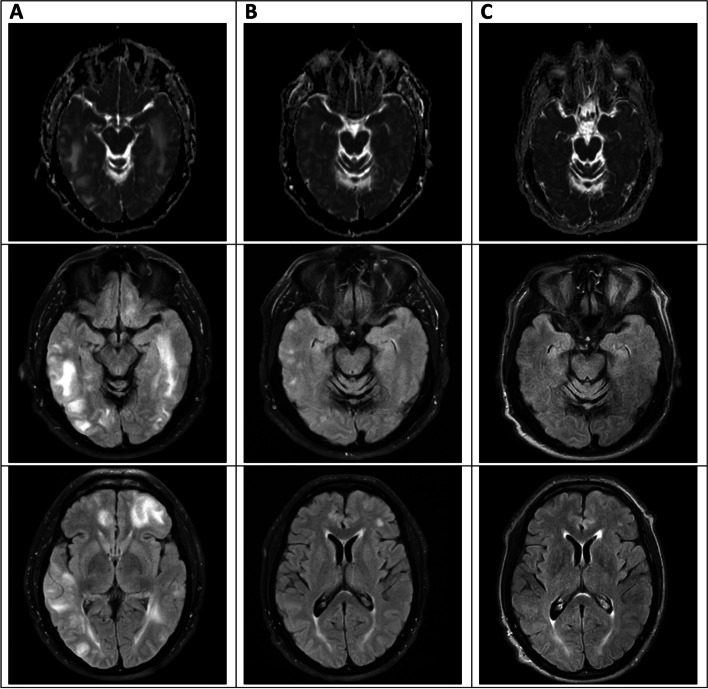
MRI sequences related to patient’s PRES. Top row Diffusion, Middle and Bottom Row FLAIR (A) Initial Presentation (B) 1-month after stopping Mepolizumab (C) 6-months after stopping Mepolizumab. Underlying white matter edema. There is also an unusual pattern of associated susceptibility artifact, which might imply a process that is hemorrhagic. In all sequences assessed, this patient’s scan is notably improved
